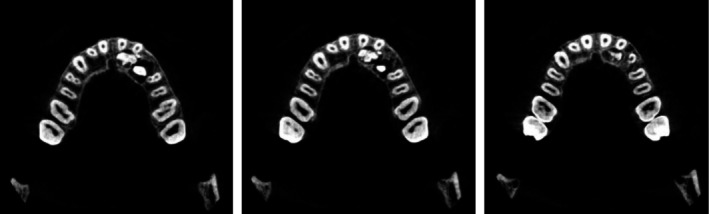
CBCT sections showing the presence of the odontoma and its proximity to permanent teeth.
